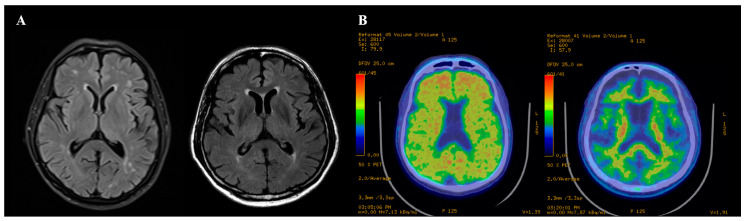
The representative images. (A) The brain MRI. The brain MRI images of the patients with amyloid burden (Left) and without amyloid burden (Right) were unremarkable. (B) The 18F-fluorbetaben PET. The left image showed significant amyloid plaque deposition in the cerebral cortex. The right image revealed a negative finding.
